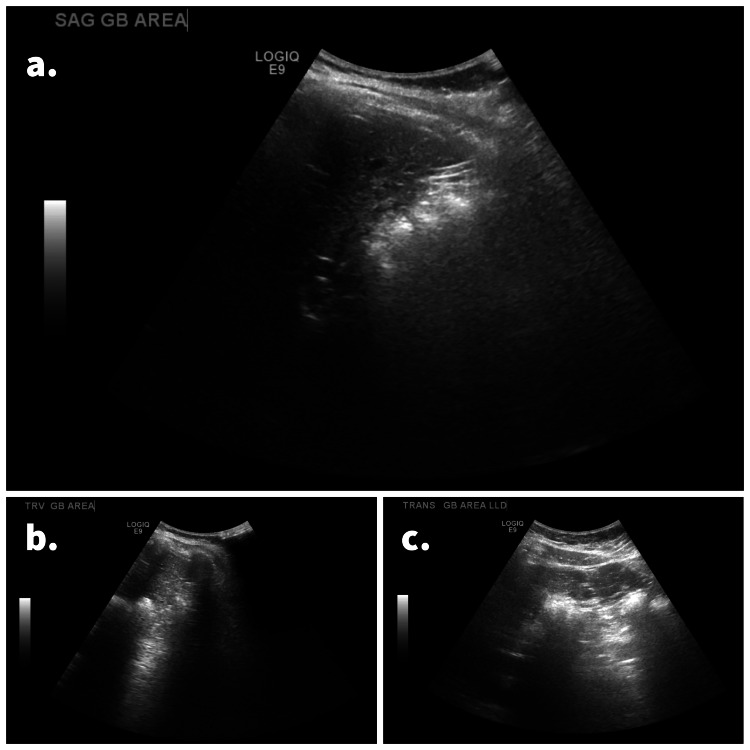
RUQ ultrasound. This was reported as a non-visualized gallbladder possibly due to a contracted gallbladder or stones filling the gallbladder leading to significant shadowing. (a) A sagittal view of the expected gallbladder location. (b and c) Alternative views of the anticipated gallbladder location. RUQ: right upper quadrant.
